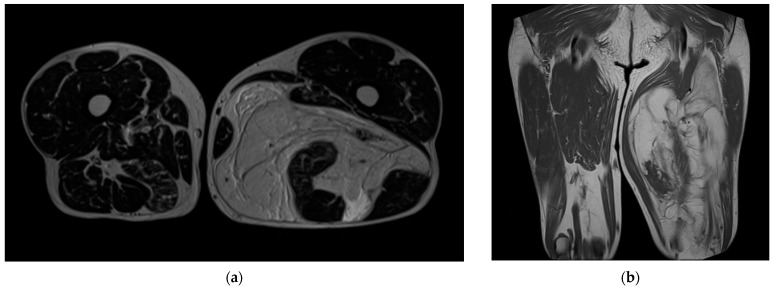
MRI images of the left thigh: (a) axial view; (b) coronal view.
